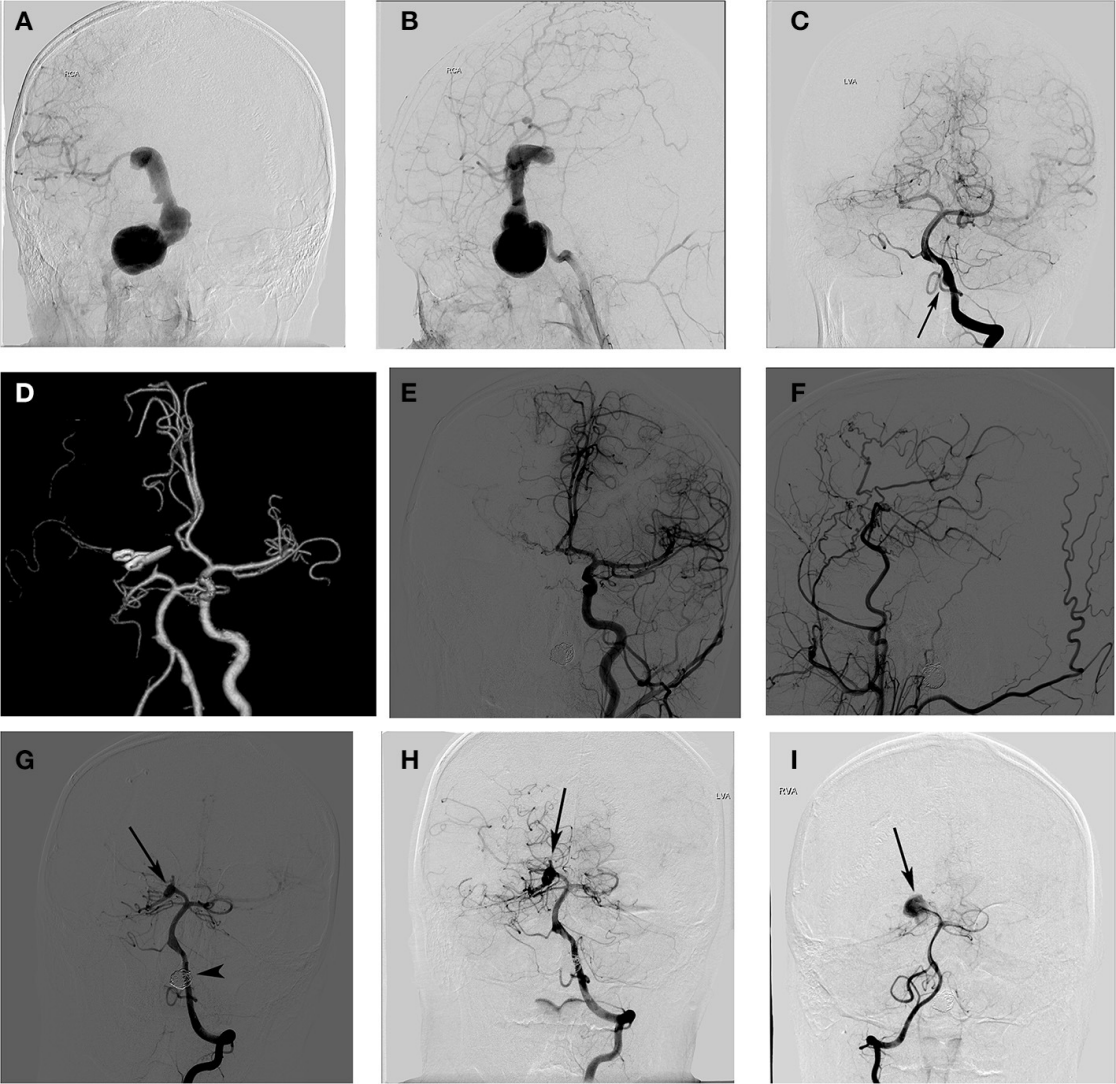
A schoolboy presented with blurred vision and headache. The right internal carotid artery (ICA) angiogram shows a giant ICA aneurysm (A,B), and the left vertebral artery (LVA) angiogram shows a dissecting aneurysm (C). Postoperative CT angiography showed complete obliteration of the aneurysm (D). The angiograms of the left (E) and right ICA (F) 2 years later showed no recurrent aneurysm and a good filling of the distal middle cerebral artery (MCA) branches via the bypass. Anterior projections of the left VA angiogram (G) showed complete occlusion of the left VA aneurysm (arrowhead) and a *de novo* aneurysm formation of the right posterior cerebral artery (PCA) (arrow). A follow-up angiogram of the right PCA showed no aneurysm enlargement 18 months after it was detected (H) and a significant aneurysm enlargement 5 years after it was detected (I).
